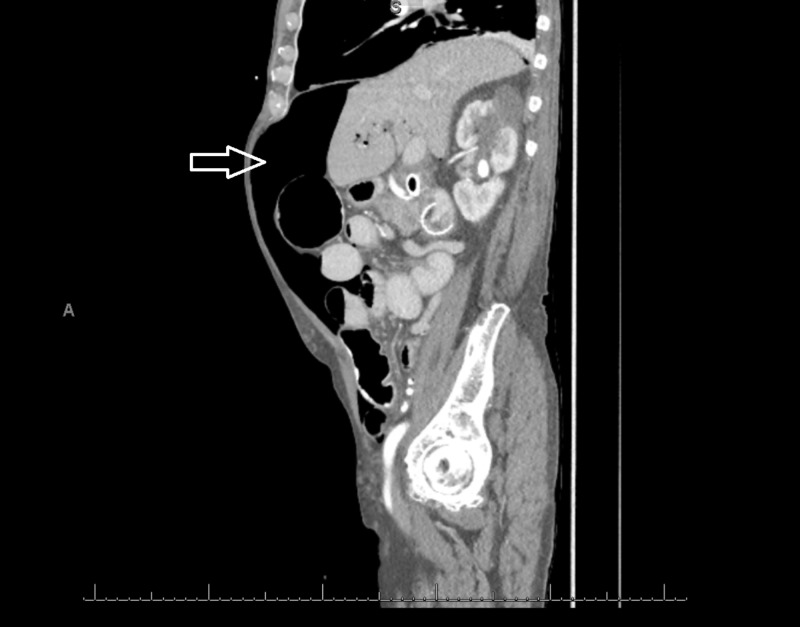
Sagittal CT scan of the patient on admission showing free air in the peritoneal cavity (solid arrow) CT: computed tomography
